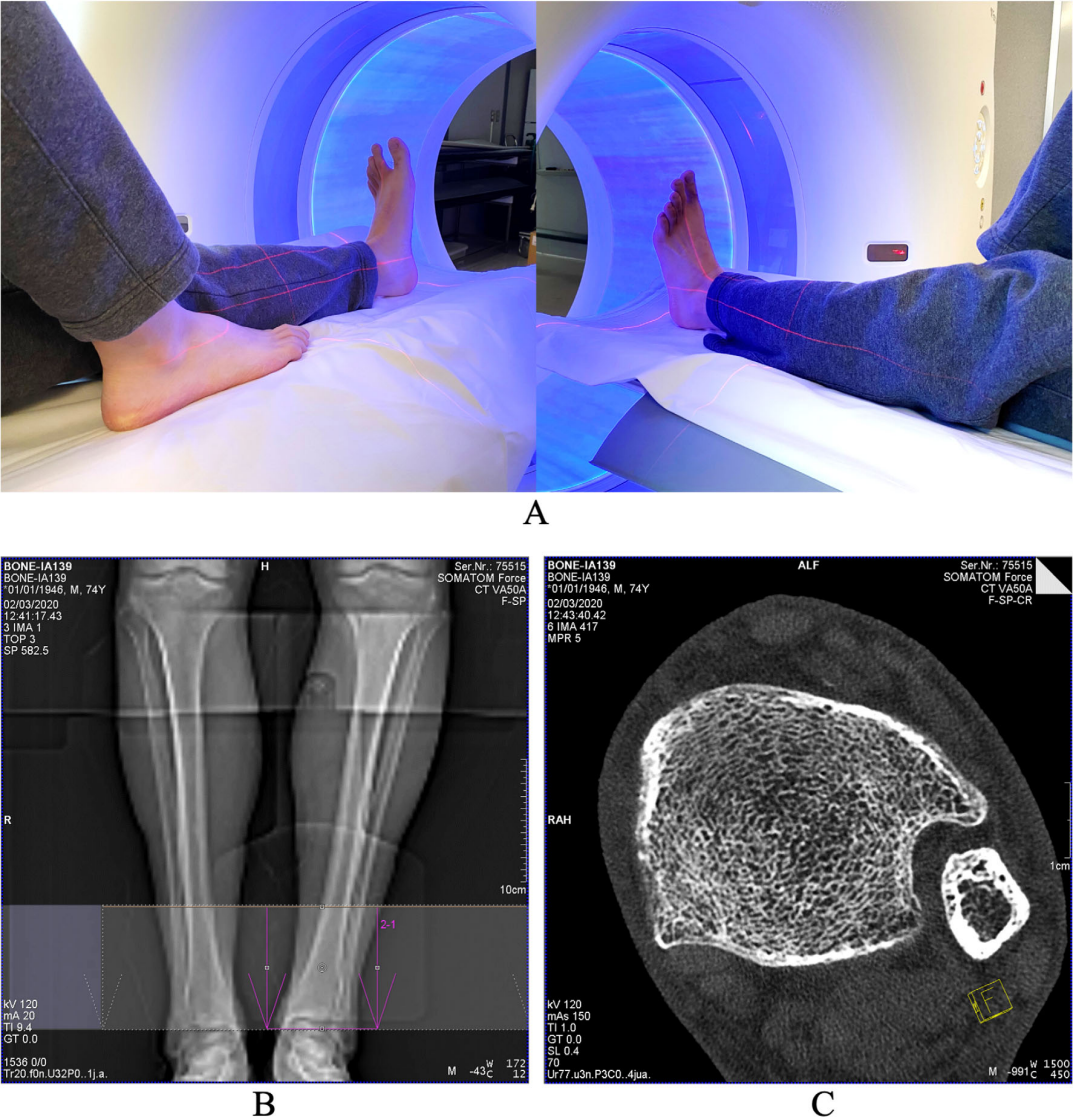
Human ankle scan setup on a Siemens FORCE CT scanner. (*A*) Positioning of the ankle. Tibial axis is aligned with the scanner center using laser rays. This alignment step is important to achieve the highest image resolution. (*B*) Positioning of the field of view (FOV) on an anterior–posterior projection CT scout scan. The distal tibial end plateau is included in the FOV, which is used to determine different tibial locations for region of interest selection during analysis. (*C*) An axial image slice from the reconstructed CT scan data.
